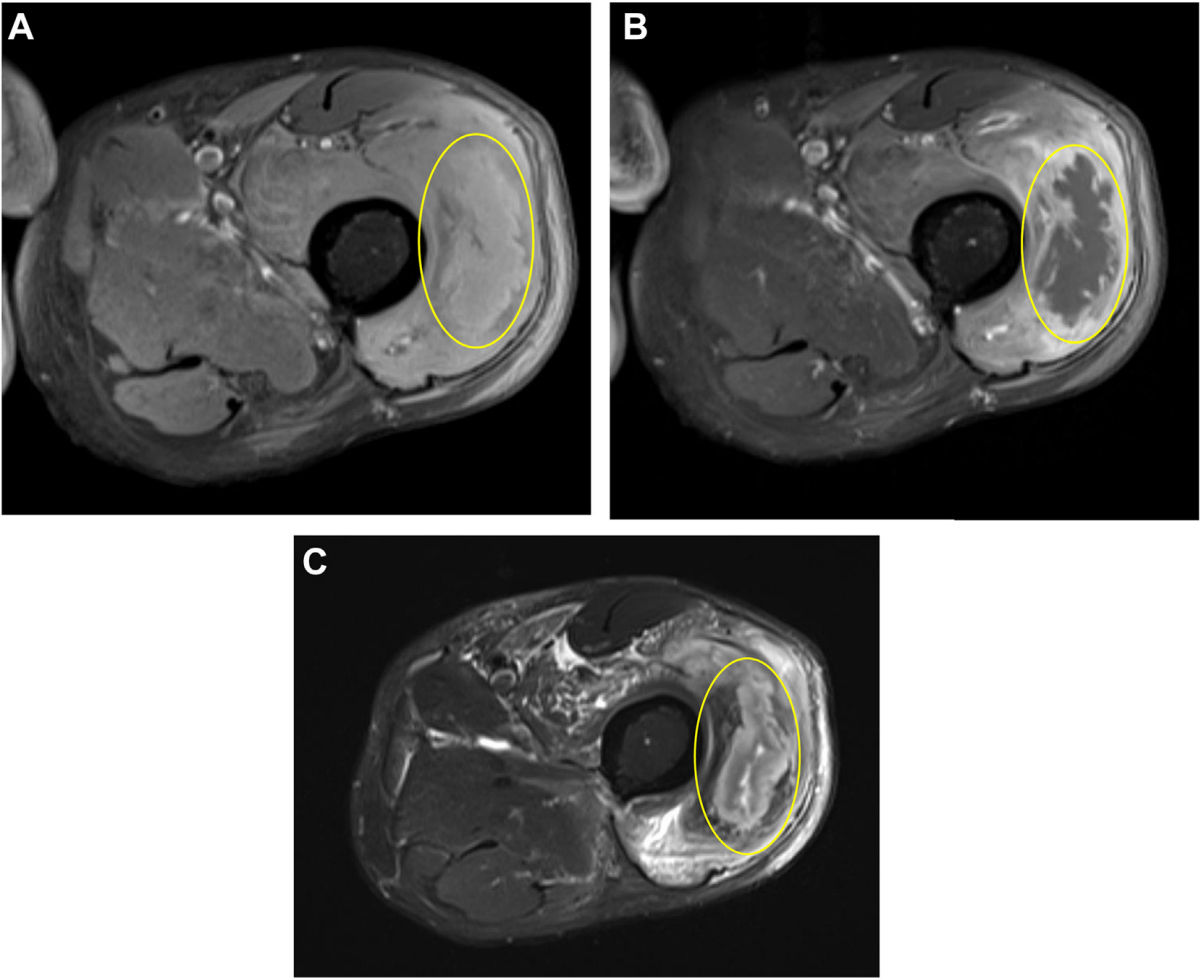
Magnetic resonance imaging of the left thigh depicting diabetic myonecrosis – large complex intramuscular fluid collection within the anterior compartment, which is internally nonenhancing with mildly T1 hyperintense and mixed T2 signal characteristics, consistent with myonecrosis. Also seen is the surrounding nonorganized, multifocal muscular, and soft tissue edema and enhancement, reflecting myositis. *A,* Precontrast T1 fat saturated (FS) image L thigh. *B,* Postcontrast T1 FS image L thigh. *C,* Axial T2 FS image of left thigh. The yellow circle highlights the areas of diabetic myonecrosis.
